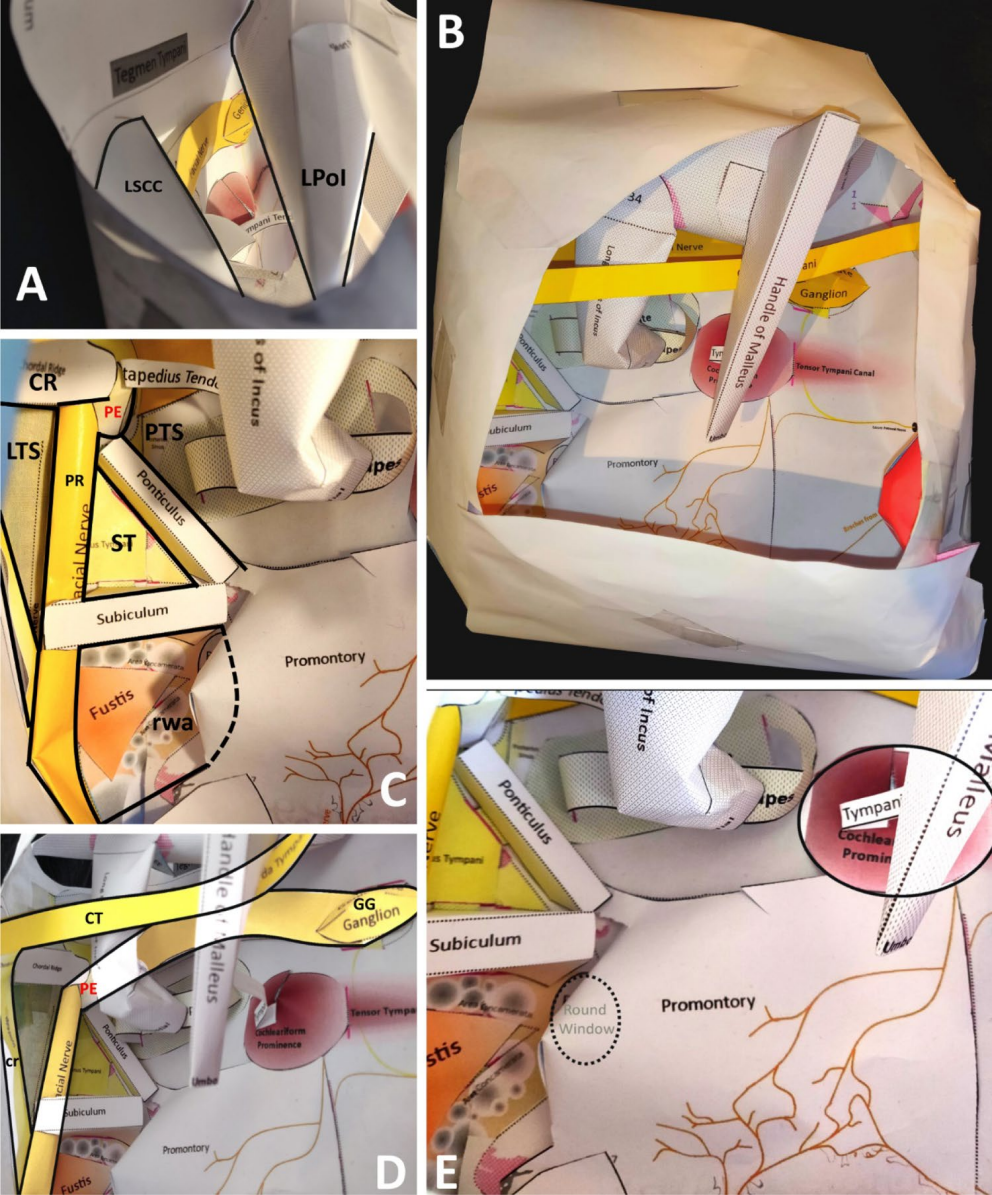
Images of the papercraft model. a View of the model via the aditus ad antrum (*LSCC* lateral semicircular canal, *LPoI* long process of incus). b View of the model representing a lateral view of a right middle ear, with ossicles in situ. c The retrotympanum (*CR* chordal ridge, *PE* pyramidal eminence, *PTS* posterior tympanic sinus, *LTS* lateral tympanic sinus, *PR* pyramidal ridge containing facial nerve, *ST* sinus tympani, *rwa* round window area). d Highlighted facial nerve (*CT* chorda tympani, *GG* geniculate ganglion, *PE* pyramidal eminence). e View of the mesotympanum. The cochleariform prominence and round window are highlighted
